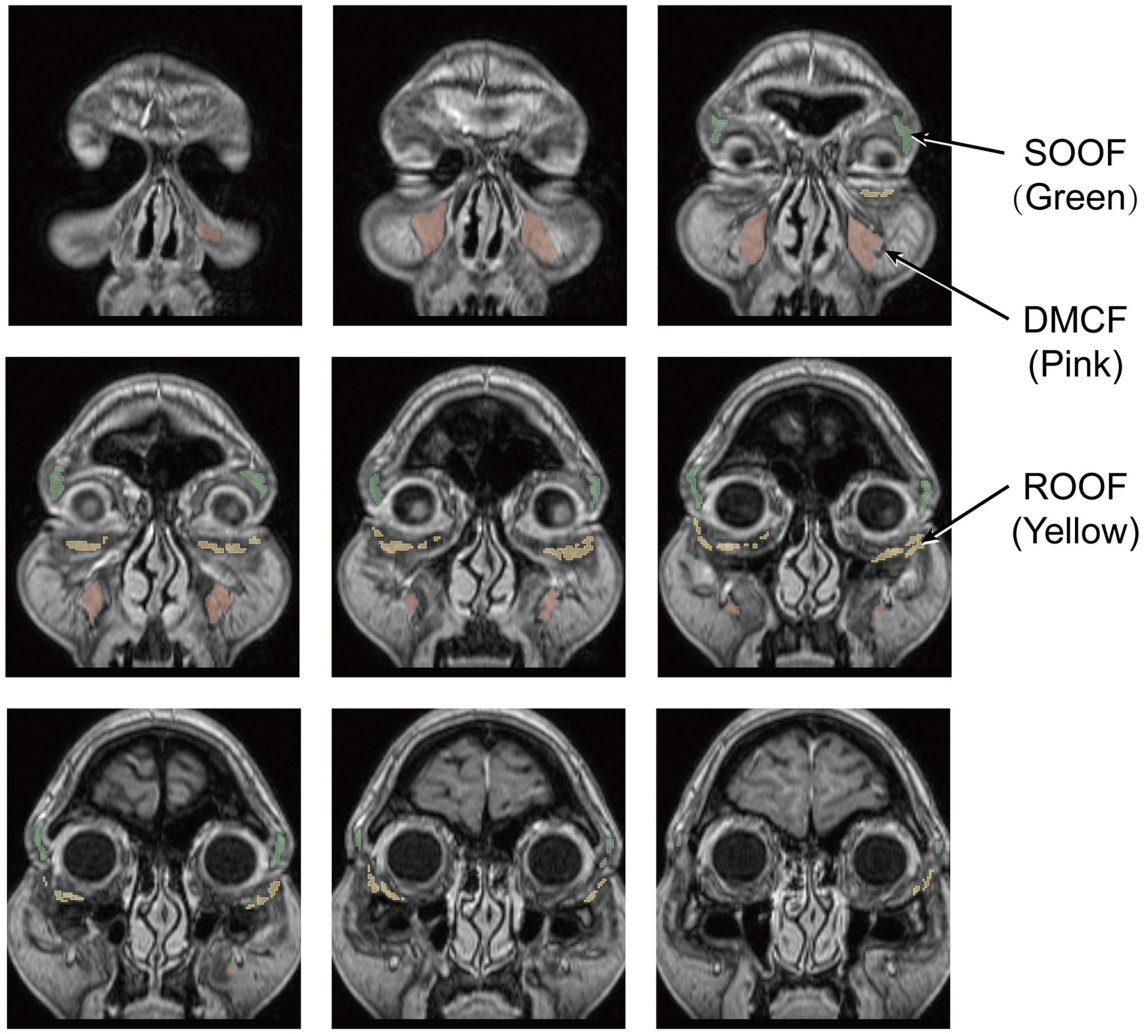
Consecutive coronal T1-weighted images of the face and three ROIs: ROOF (green), SOOF (yellow), and DMCF (pink).
